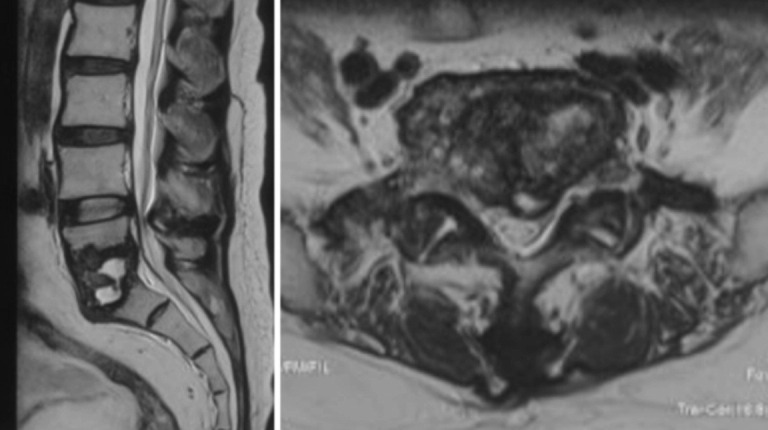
Magnetic resonance imaging (MRI) T2 weighted sagittal view showed cystic lesions without adjacent marrow oedema under the irregular end plates of L5 and S1.
